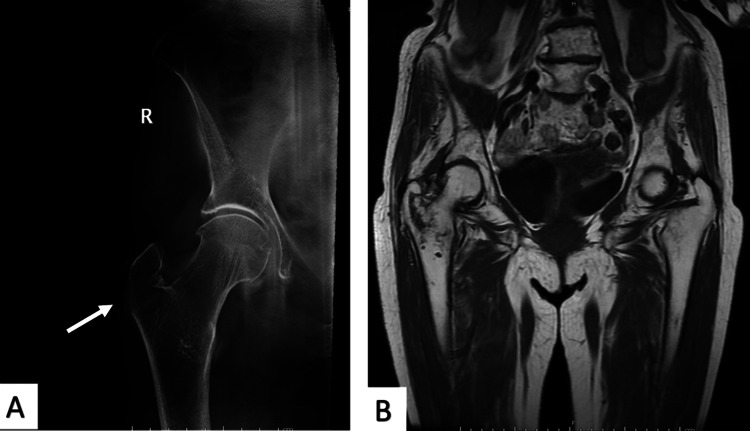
An 85-year-old female patient tripped on furniture and presented with pain and non-weight-bearing. Plain radiographs did not show an obvious fracture. There is a small undisplaced fracture of the right greater trochanter on the tomogram (A, arrow) and MRI (B).
